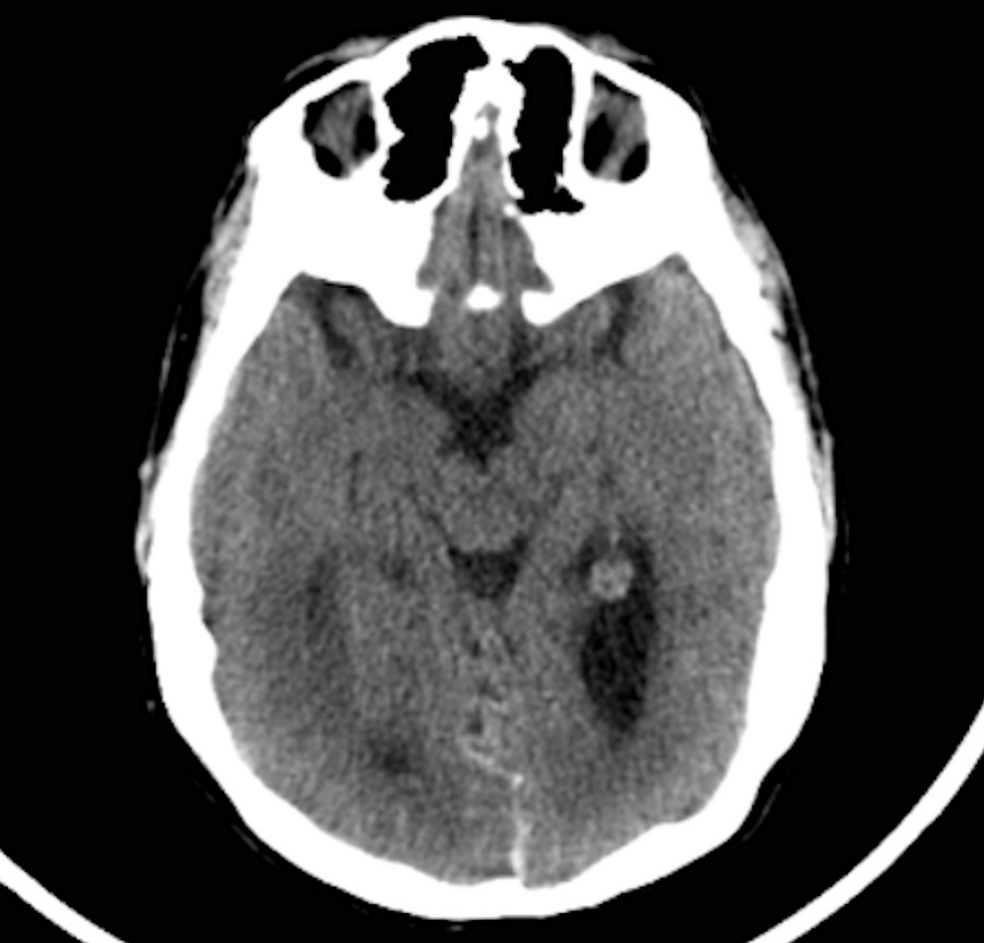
Non-contrast CT scan of the brain showing the absence of blood in the basal cisterns
